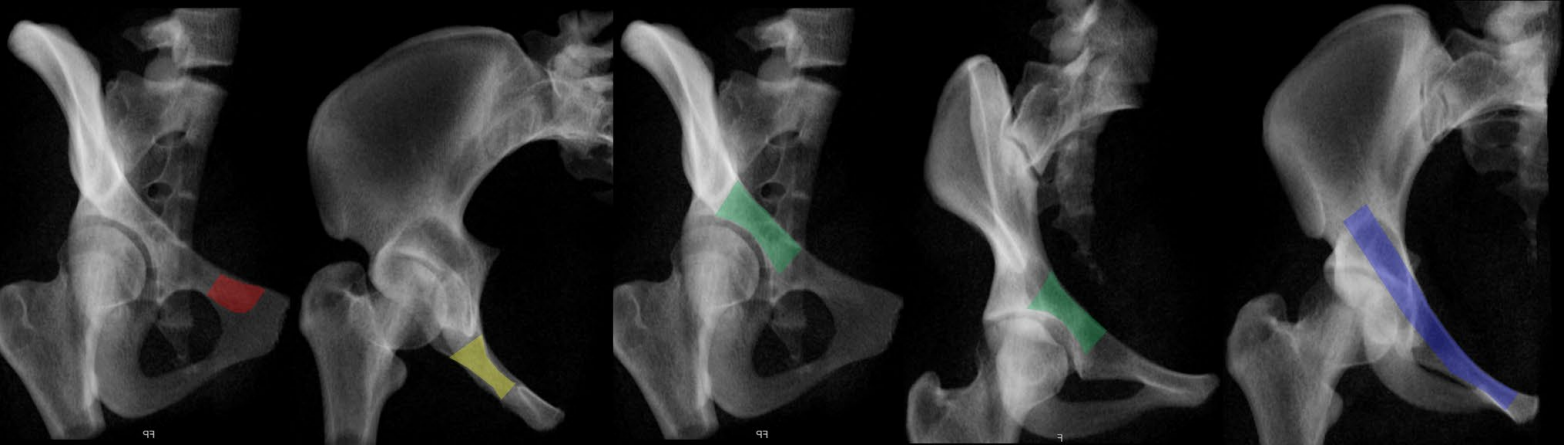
Radiographic sequence for retrograde superior ramus screw or antegrade anterior column screw: COOO, hyper-Inlet, COOO, COOI and pelvic Inlet view with corresponding areas for optimal guide-wire analysis (colored)
